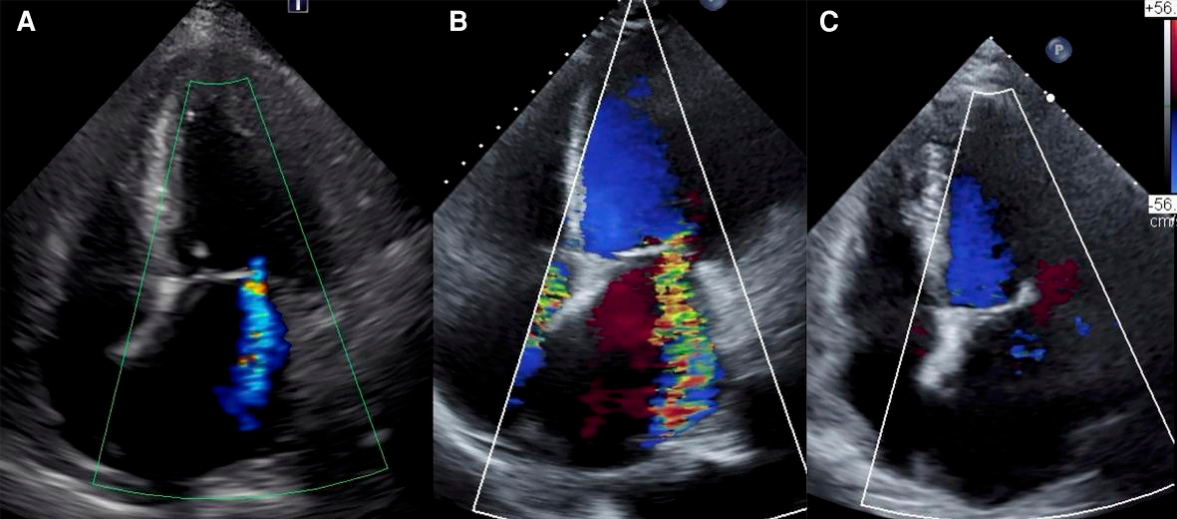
Echocardiographic findings. (*A*) Transthoracic echocardiography (TTE) at 1st admission to our hospital revealed left ventricular ejection fraction (LVEF) of 35% and mild mitral regurgitation (MR). (*B*) TTE before mitral transcatheter edge-to-edge repair of mitral valve (M-TEER) procedure revealed LVEF of 33% and severe MR. (*C*) TTE 3 months after M-TEER revealed LVEF of 44% and mild MR.
